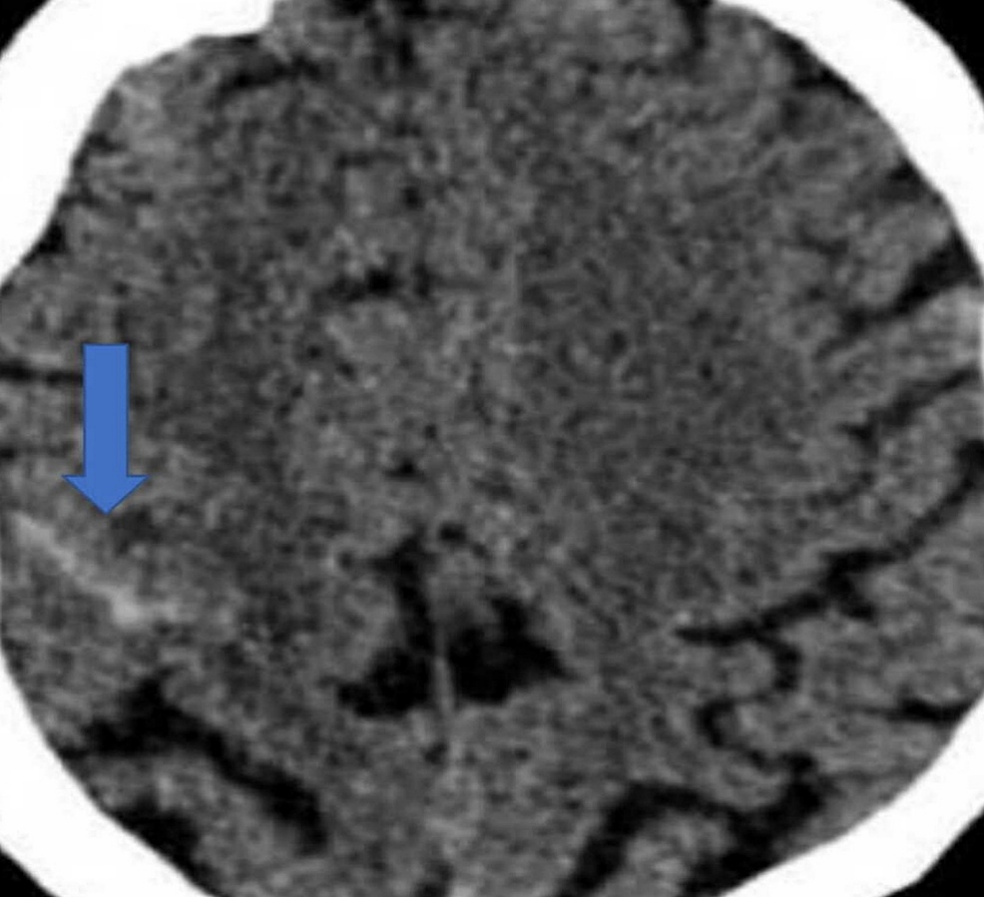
Non-contrast CT head axial showing subarachnoid haemorrhage (arrow) in the right frontal, superior parietal regions
